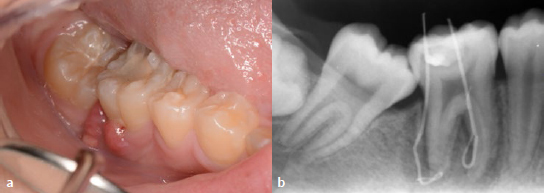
Preoperative examination of the tooth 46. a) The patient complained of gingival inflammation. b) Preoperative radiograph with inserted gutta-percha in sinus tracts.
